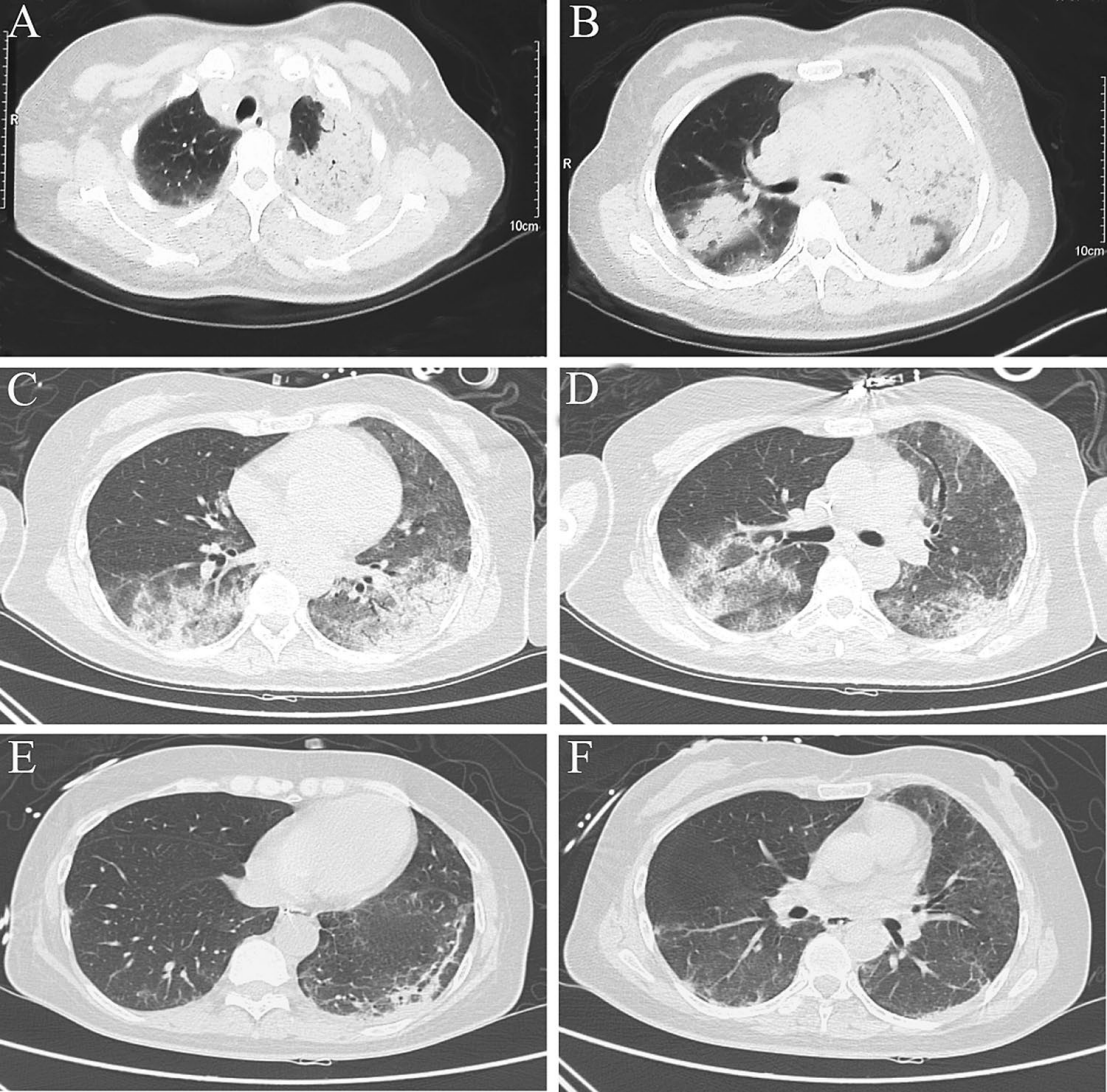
Serial chest computed tomography (CT) scans of a 49-year-old woman with severe psittacosis pneumonia. The initial CT scan (10 days after onset) shows air-space consolidation with inflammatory exudation in the superior lobe of the left lung and inferior lobe of the right lung (a, b). CT scan (19 days after the onset) shows that the consolidation area gradually decreased following treatment with extracorporeal membrane oxygenation (c, d). On follow-up, the consolidation disappeared, 26 days after the onset (e, f)
